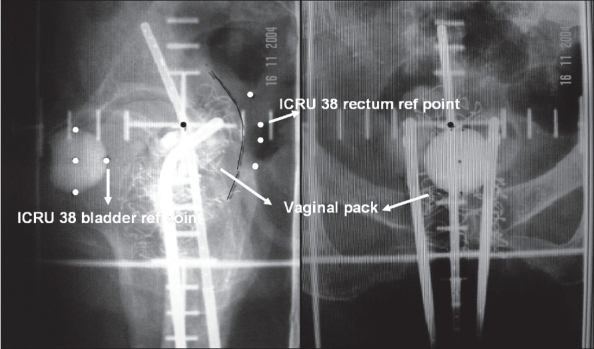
ICRU rectal and bladder reference points are marked on orthogonal radiographs. Rectal reference points are taken 5 mm posterior to the vaginal wall, which could be visualized from the radiopaque gauze packing
